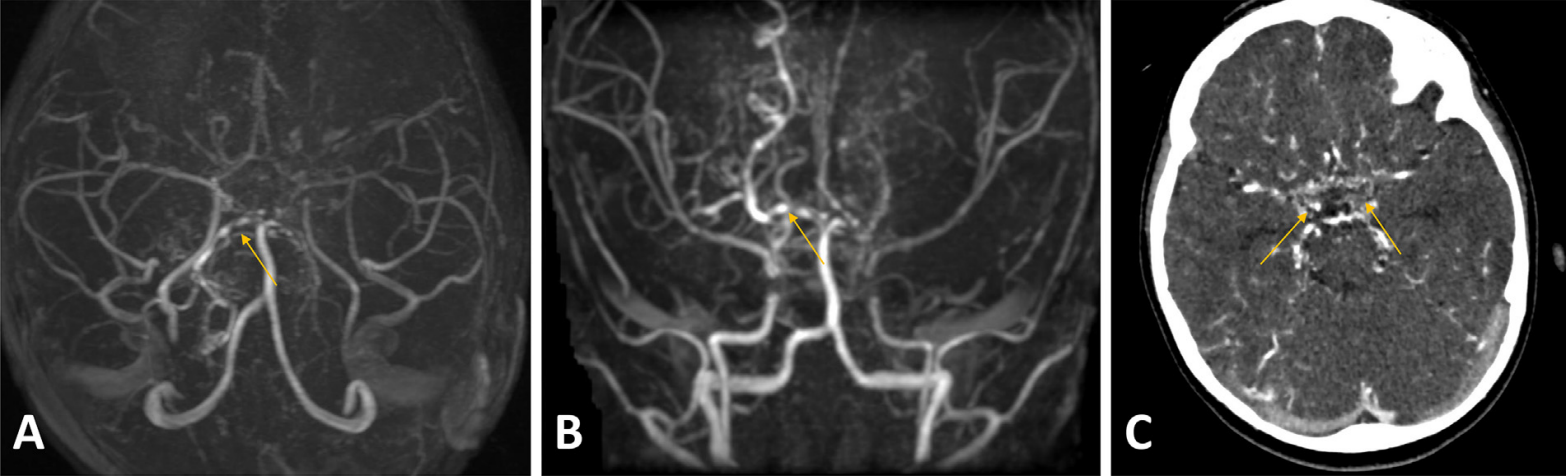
MRA 3D axial (A) and coronal (B), beaded appearance (arrowheads) of the circle of Willis with the formation of numerous collaterals (CTA, C arrows) demonstrating puff of smoke appearance.
